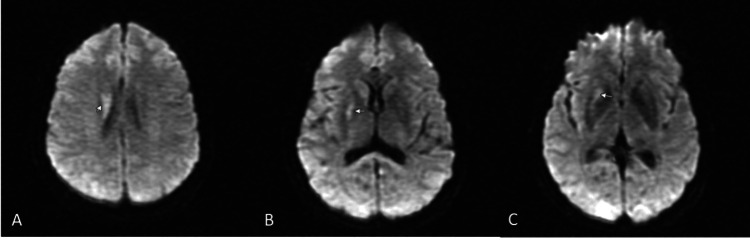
Brain MRI images with findings marked with arrows (A) A focus of increased signal intensity was noted in the body of the caudate nucleus adjacent to the body of the right lateral ventricle. This is increased in signal intensity on the T1-weighted image and low in signal intensity on the apparent diffusion coefficient (ADC) map, suggesting acute to subacute infarct. (B, C) A similar finding is visualized in the right lentiform nucleus with a mildly increased signal on the diffusion-weighted images, which is hypo-intense on the ADC map and consistent with an acute to subacute infarct.
